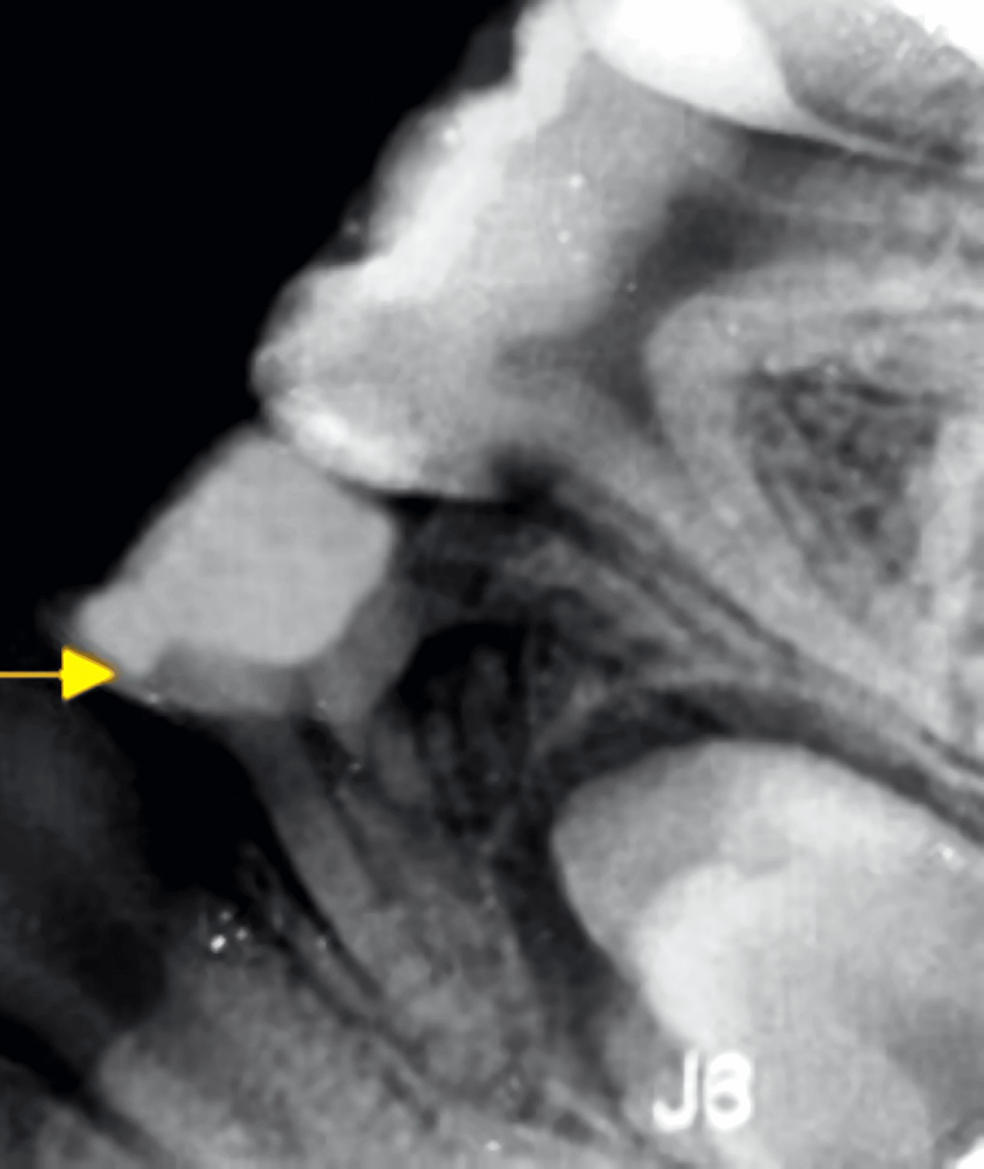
Depiction of the immediate post-operative radiograph of RE of the left deciduous mandibular first molar RE: Radix entomolaris
